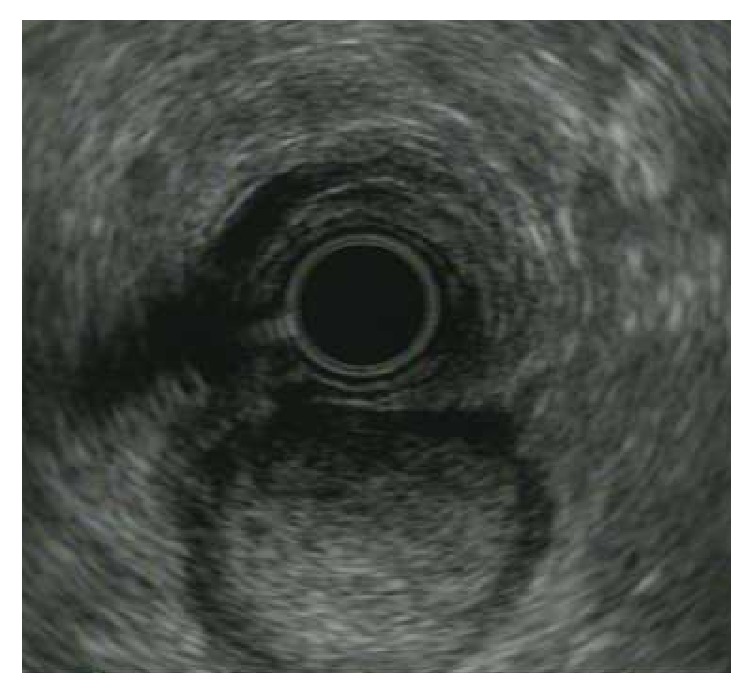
Ultrasound image of glomus tumor.
